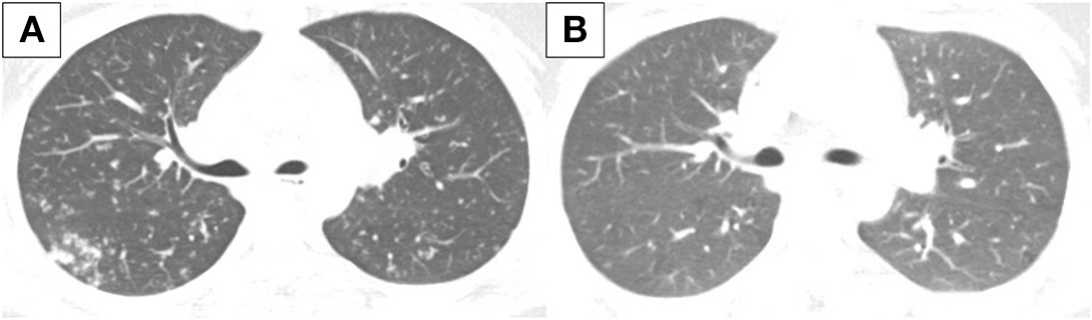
Chest CT conducted (A) at diagnosis of miliary TB and (B) after TB treatment. (A) Innumerable small discrete nodules of random distribution with tree in bud pattern are observed in both lungs. (B) There is a decrease in extent of the innumerable small nodules in both lungs. CT, computed tomography; TB, tuberculosis.
